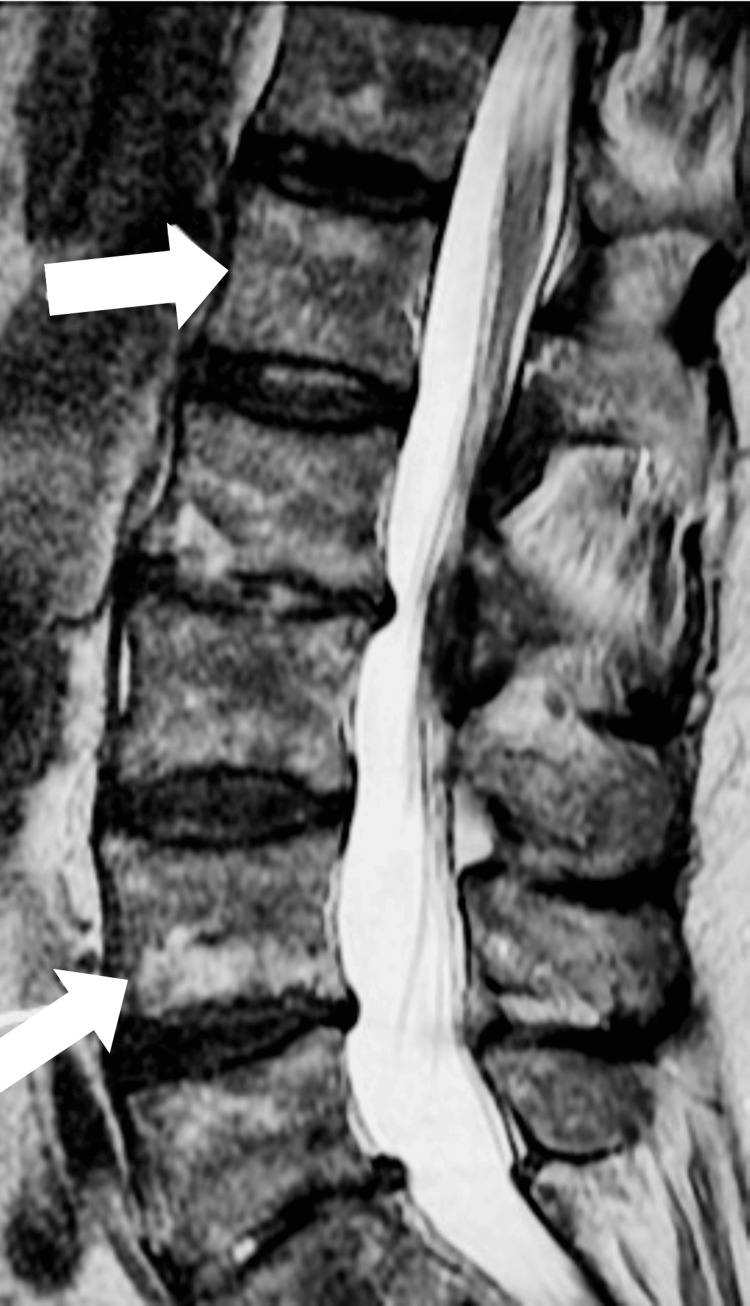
Abnormal T2 sagittal lumbar MRI demonstrating diffuse bone marrow changes and disk degeneration with disk bulges at L5/S1 and L2/3. Arrows mark the diffuse bone marrow changes MRI: magnetic resonance imaging
